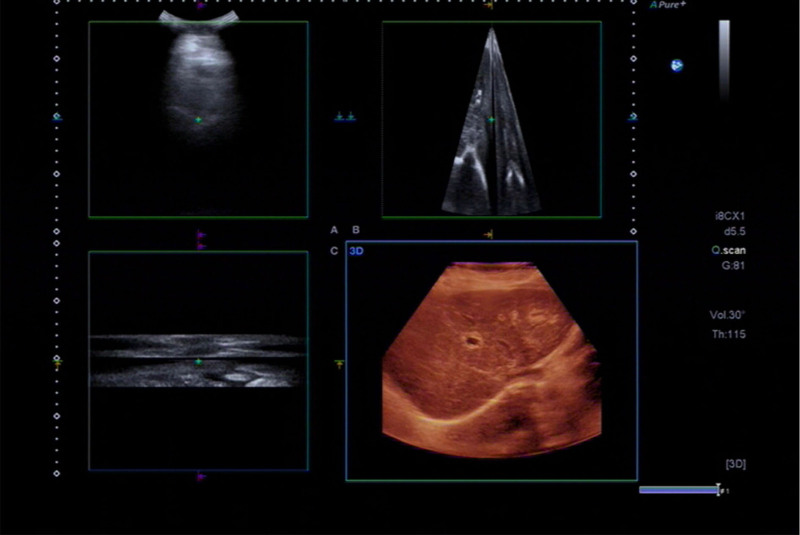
Follow-up liver ultrasonography.
